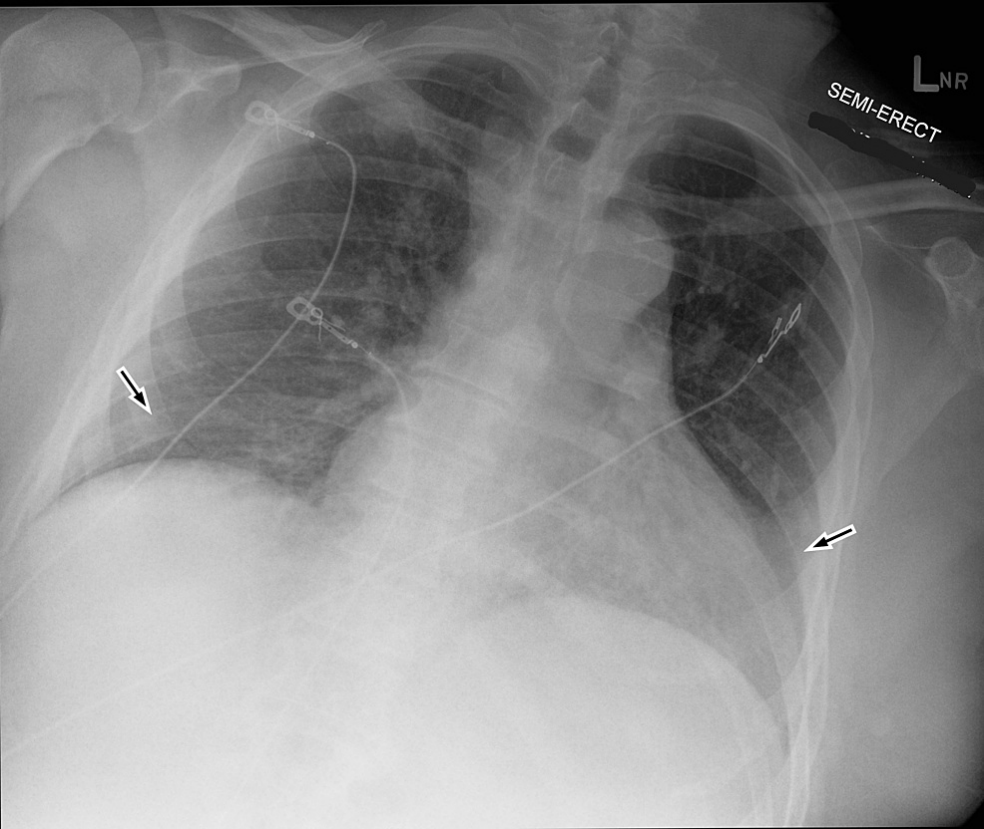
Chest radiograph during the first visit Black arrows indicate bibasilar atelectasis
